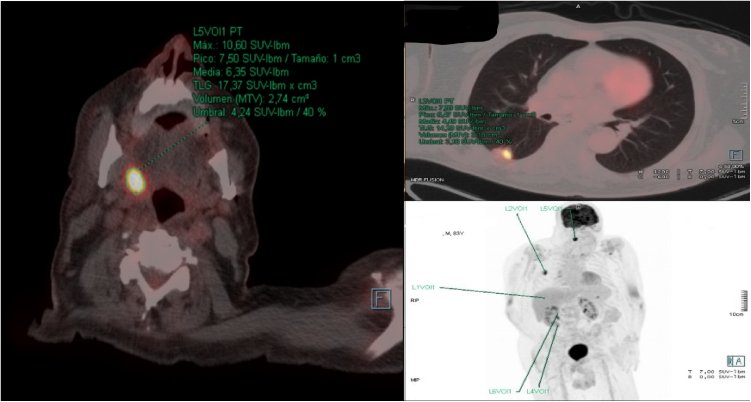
PET-CT showed an uptake of 18F-FDG in a nodule of the lower lobe of the right lung, and in the right palatine tonsil.
